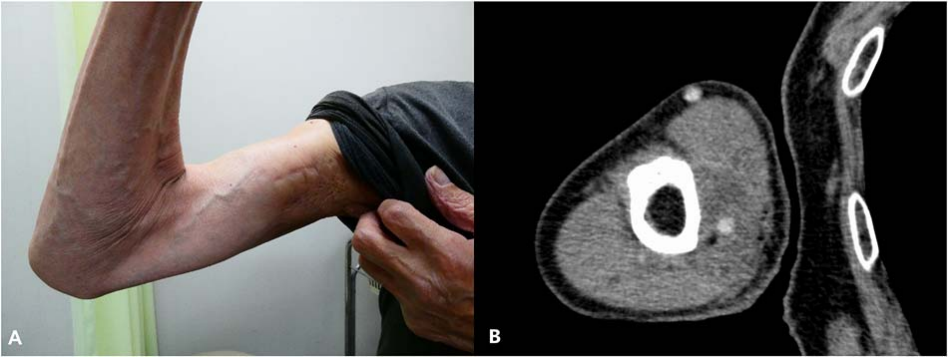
(A) The right upper arm. Mild pigmentation and atrophy of the irradiated site are shown. (B) An axial image of the contrast enhanced CT that was taken 2 years after the treatment. No local progression was noted. The circulation of the brachial artery can be seen.
